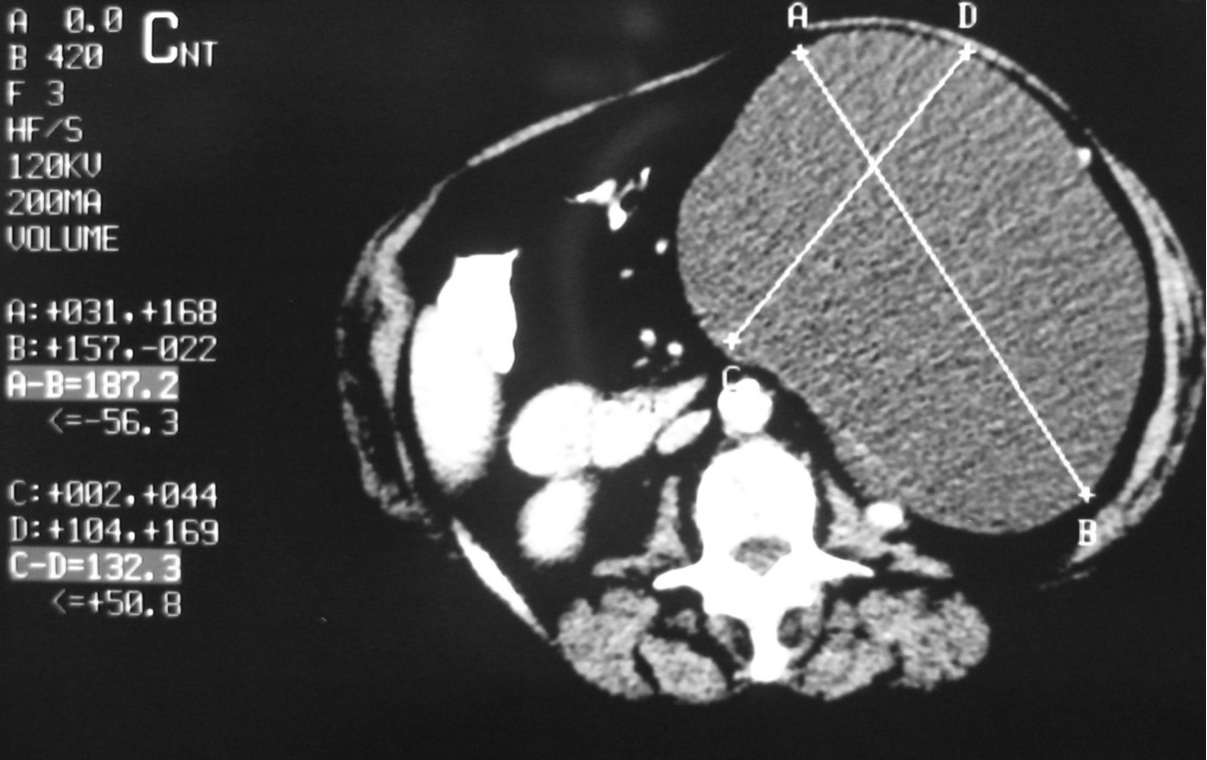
Demonstration of the maximum dimensions of the mass, on CT axial plane.
